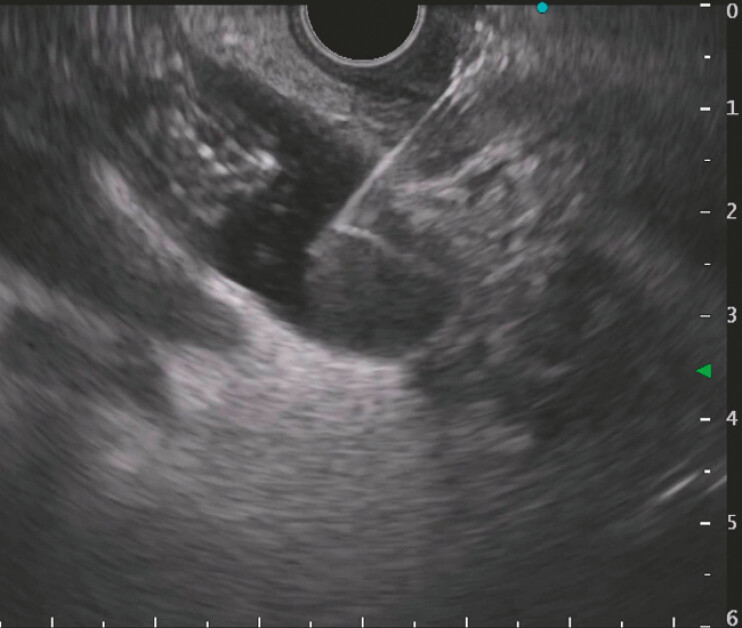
EUS-guided puncture of the distal CBD using a 19G needle. Maldeployed FCSEMS visualized intraductally. CBD, common bile duct; EUS, endoscopic ultrasound; FCSEMS, fully covered self-expandable metal stent.
